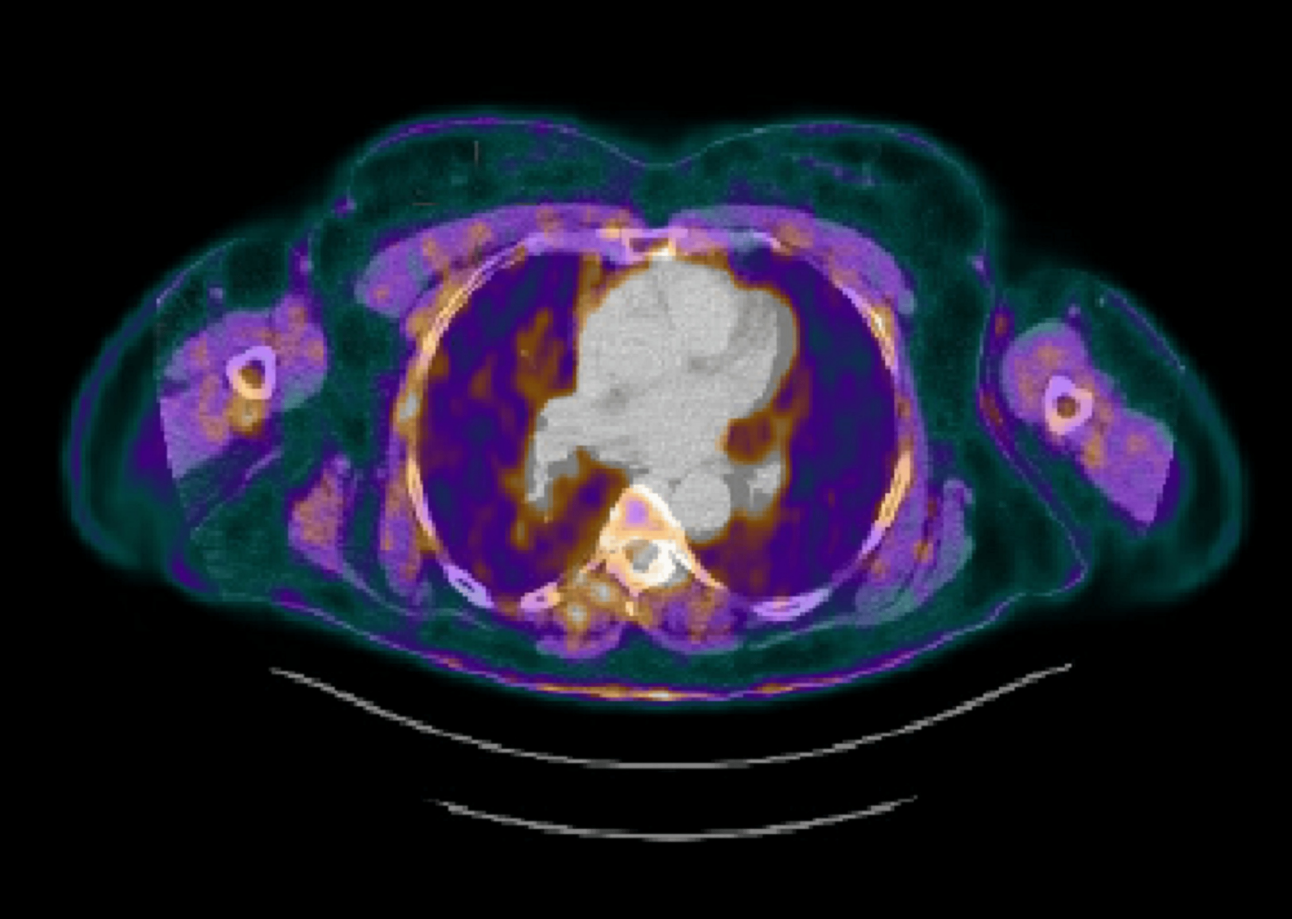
18F-FDG PET-CT after treatment, where a complete response is visible 18F-FDG PET-CT: 18F-fluorodeoxyglucose positron emission tomography-computed tomography
